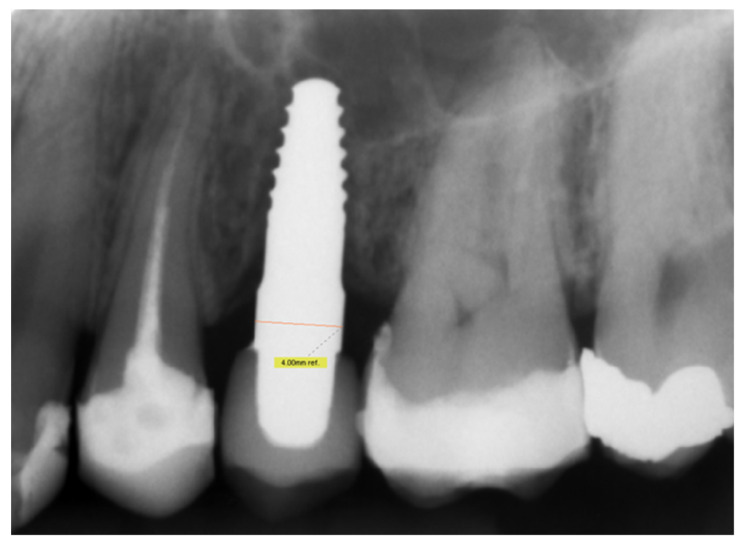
Single tooth radiograph of a 34-year-old male patient 25 months after implantation. The bone loss at the implant site was clearly visible, while the crestal bone level of the adjacent teeth was not affected.
